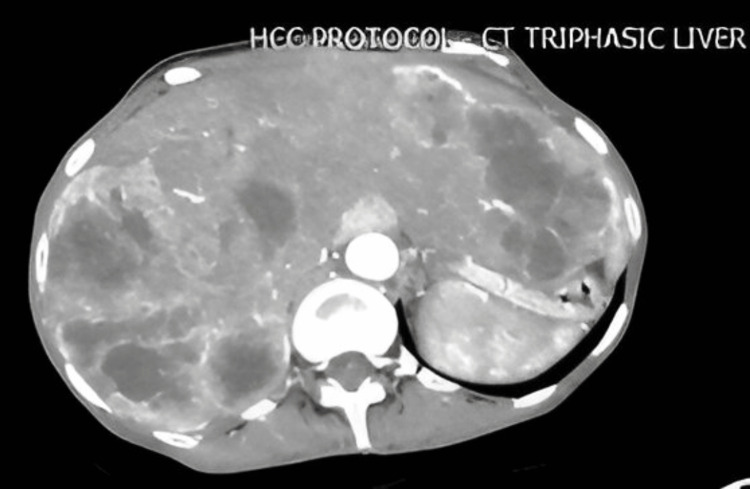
CT scan shows multiple large heterogenous and necrotic lesions in both lobes of liver. CT, computed tomography; HCC, hepatocellular carcinoma
